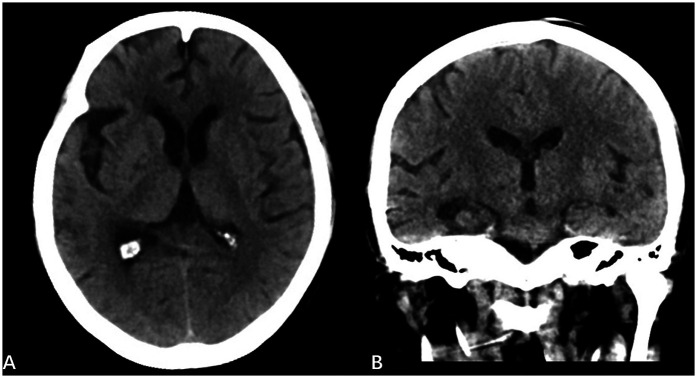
Postoperative CT scans (A) and (B) show significant reduction in: right fronto-temporo-parieto-occipital subdural hematoma,subarachnoid hemorrhage, compared to preoperative images.
